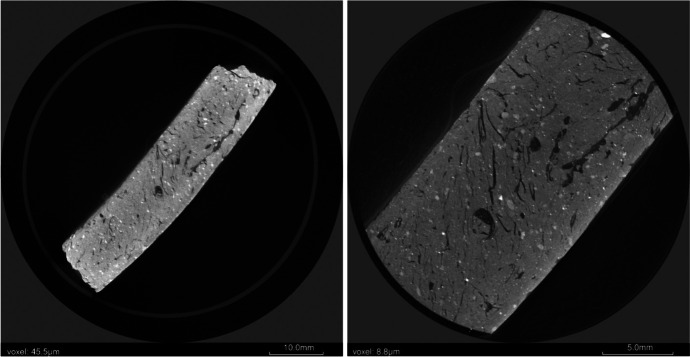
2D radiographic slices of sorghum-tempered pottery sherd. Left. Slice from full sherd scan. Right. Slice from ROI (region of interest scan
